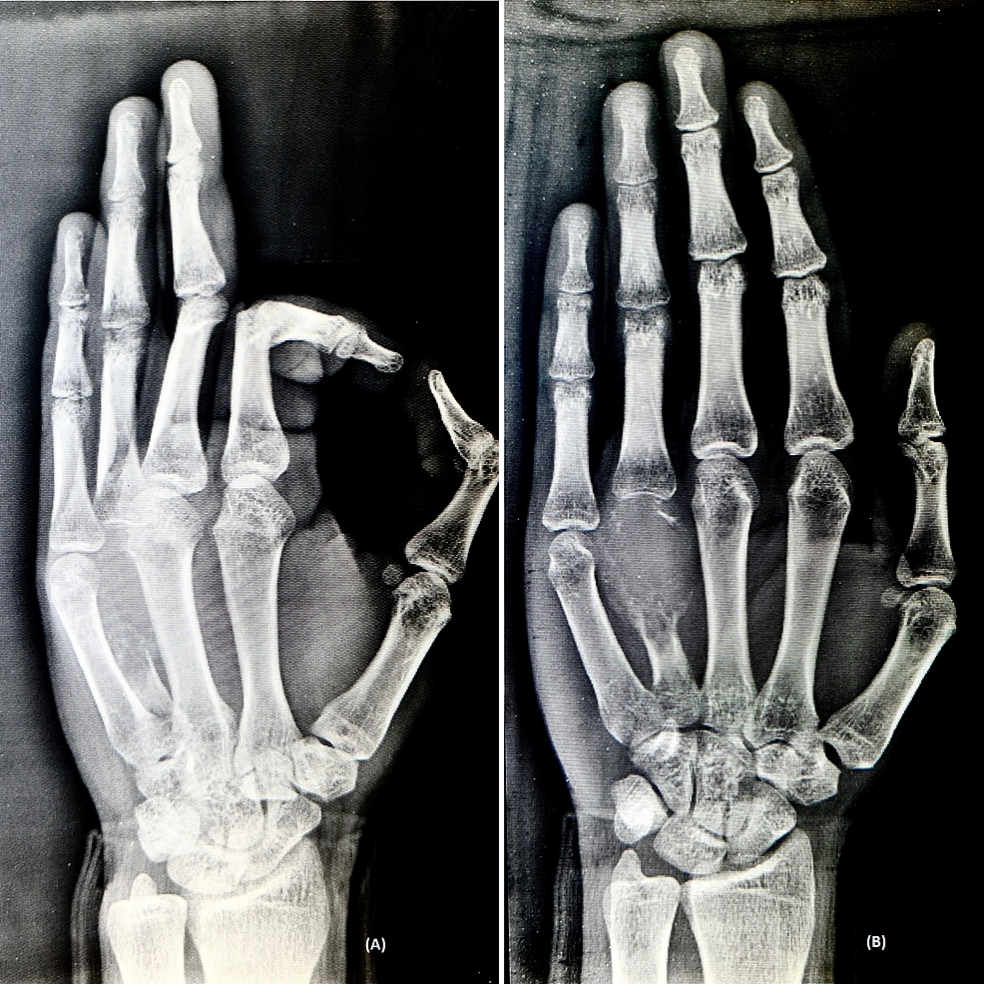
Radiograph of the left hand in A) oblique view and B) anteroposterior view.
